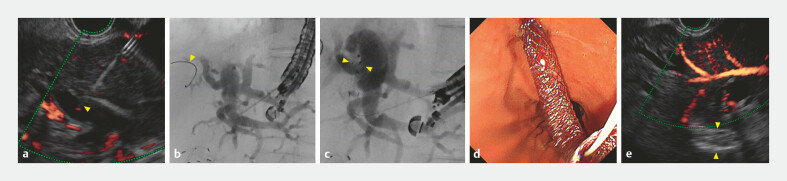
Images during the first endoscopic ultrasound-guided hepaticogastrostomy with stent misplacement.
